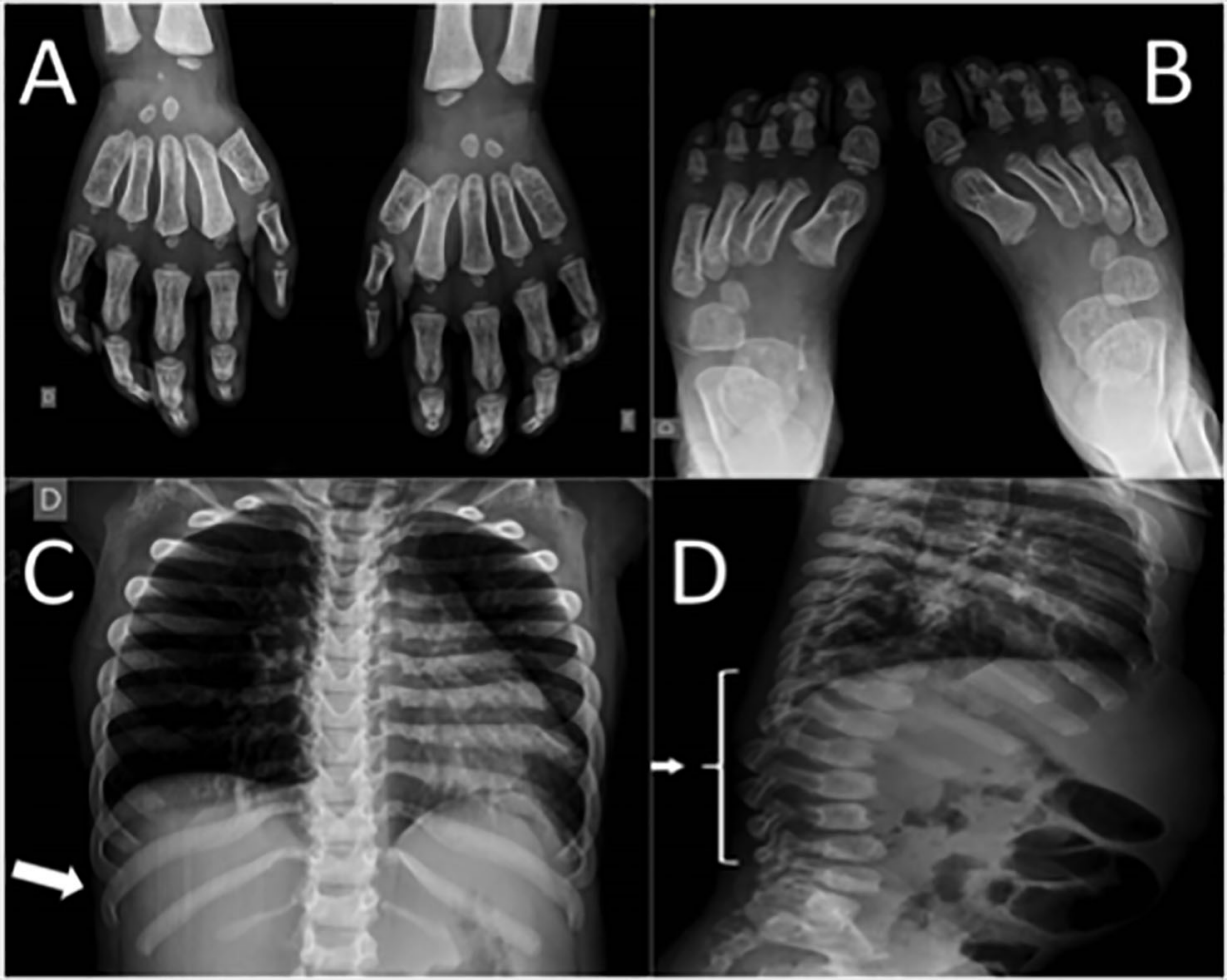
Patient's radiographic findings. (A) Proximal pointed phalanges of hands. (B) Proximal pointed phalanges of feet. (C) Widened anterior and tapered posterior ribs (arrow). (D) “Beaked vertebra” of lumbar spine (arrow).
